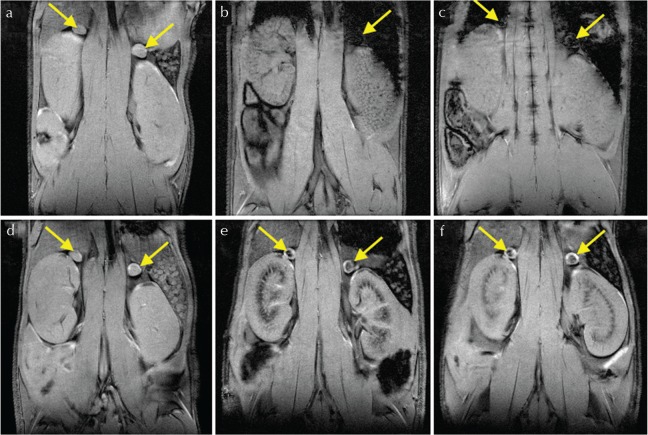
Magnetic resonance imaging (MRI) of adrenal glands before and after the intravenous administration of Resovist (a–c) and the New super paramagnetic iron oxide (SPIO) (d–f). MRIs were obtained at each time point: before (a, d), 1 week post (b, e), 4 weeks post (c, f) the administration. Yellow arrows indicate adrenal glands.
